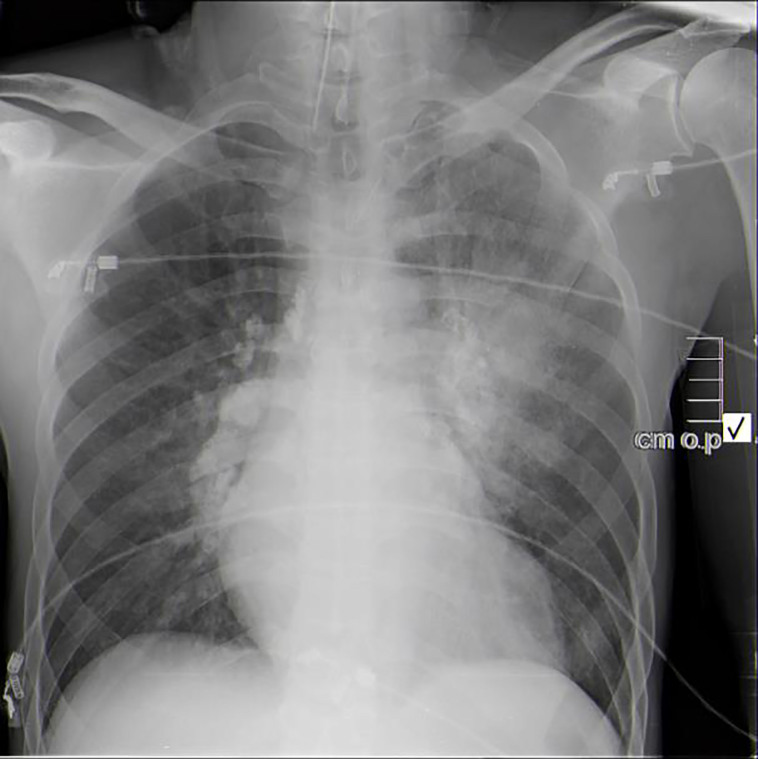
Anterior posterior chest radiograph demonstrates unilateral (left sided) opacities.
